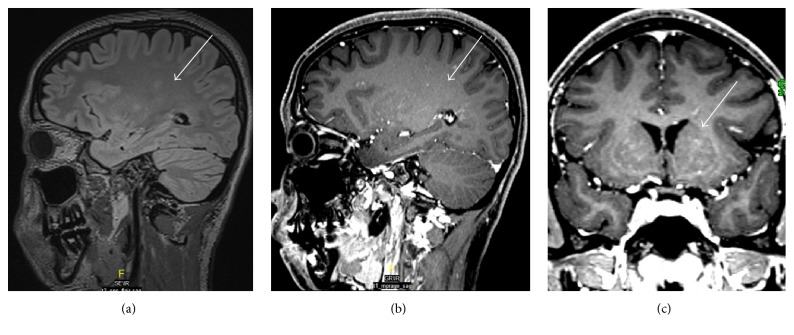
(a) Sagittal T2/FLAIR, (b) sagittal, and (c) coronal T1 weighted MRI after IV gadolinium based contrast medium injection, of the brain, showing white matter ill-defined faint bright intensity (arrows in (a)) and characteristic punctate and linear enhancement in white matter and basal ganglia (arrows in (b) and (c)), respectively.
